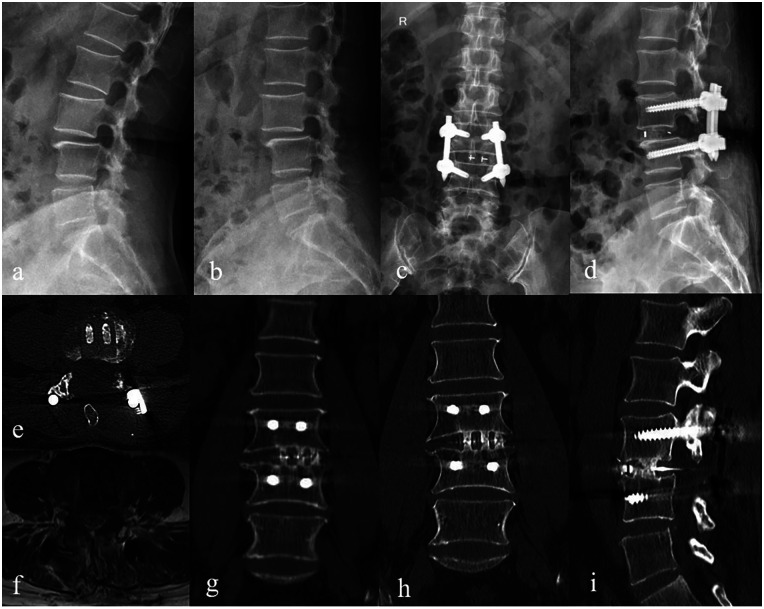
(a,b) A 58-year-old female patient with refractory low back pain and intermittent claudication, exhibiting L3 degenerative spondylolisthesis and lumbar instability on x-ray. (c–f) Postoperative imaging (x-ray, CT, and MRI axial views) at 2 days post-UBE-LIF, demonstrating satisfactory internal fixation and two cages in position. (g) CT at 3 months post-surgery showing no solid fusion at L3-L4. (h,i) CT at 6 months post-surgery confirming solid fusion at L3-L4.
